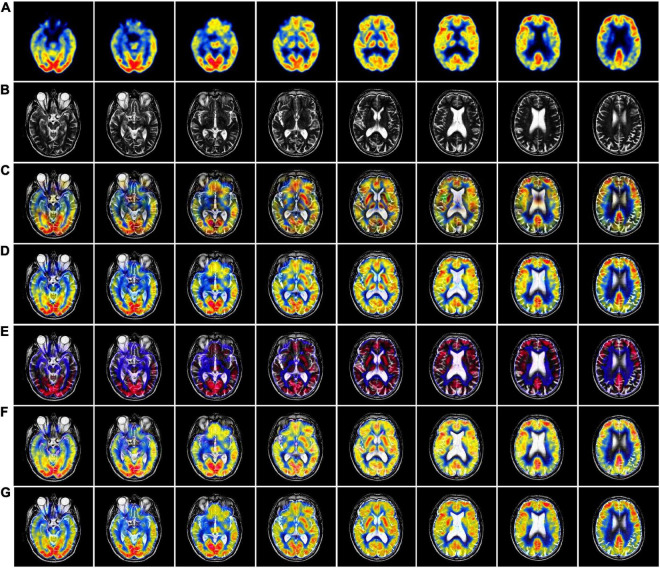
MRI-PET fusion images of five methods in Paras2. (A) Positron emission tomography (PET) source image; (B) magnetic resonance imaging (MRI) source image; (C) nonsubsampled contourlet (NSCT) fusion result; (D) non-subsampled shear wave transform (NSST) fusion result; (E) guided filter ng fusion (GFF) fusion result; (F) Laplacian redecomposition (ReLP) fusion result; (G) is the fusion result of the proposed method.
